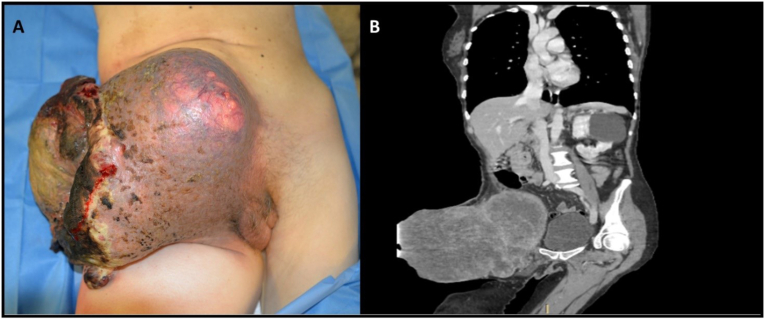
A: Pre-treatment Giant Melanoma; B: Pre-treatment CT imaging Giant Melanoma.
